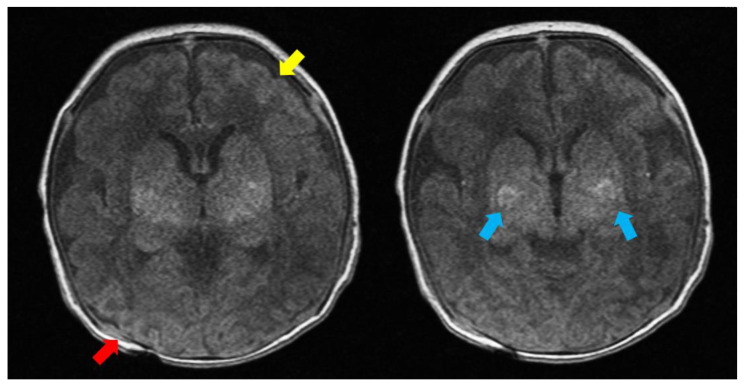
Brain MRI T1-weighted axial images on day 3 showing multifocal hypoxic-ischemic lesions in both putamen and thalami (blue arrows), right occipital (red arrow) and left frontal (yellow arrow) subcortical white matter.
